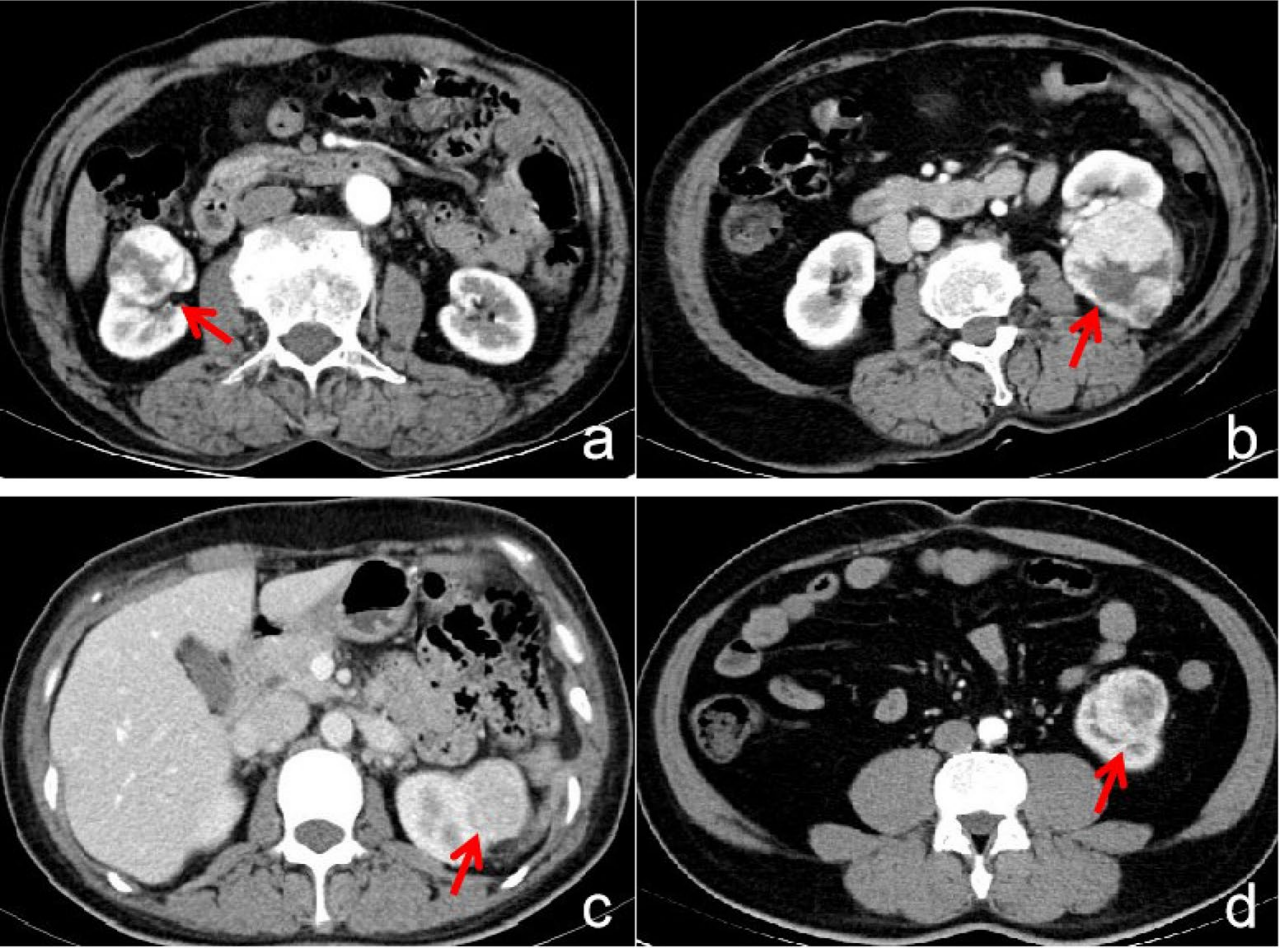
(a) A 52-year-old man with ccRCC, the CT image of CMP shows the sign of pseudocapsule (arrow). (b) A 51-year-old man with ccRCC, the CT image of NP shows cyst region in the mass (arrow). (c) A 39-year-old woman with fp-AML, the CT image of NP shows the homogeneous enhancement pattern (arrow). (d) A 55-year-old man with ccRCC, the CT image of CMP shows the heterogeneous enhancement pattern (arrow).
